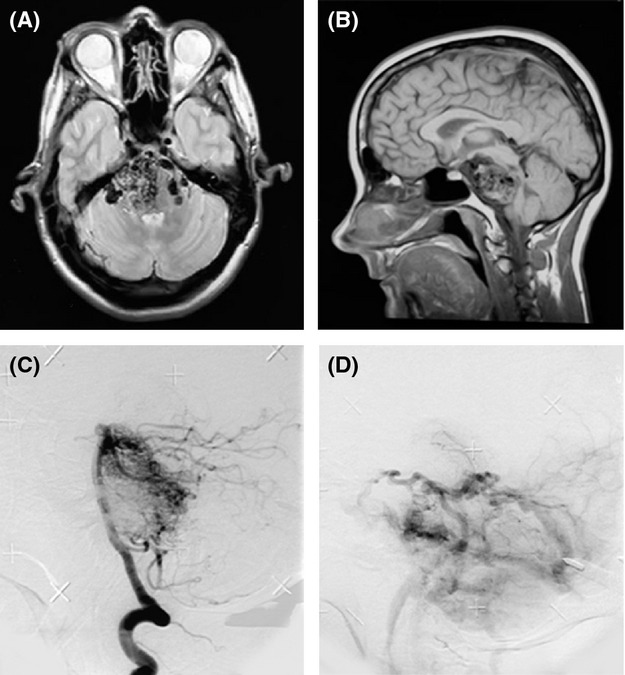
AVM located in right pons. (A) Axial T2-weighted MRI brain. (B) Sagittal T1-weighted MRI brain. (C) Digital subtraction arteriogram, vertebral artery injection, lateral view, arterial phase. (D) Digital subtraction arteriogram, vertebral artery injection, lateral view, venous phase. AVM, arteriovenous malformations; MRI, magnetic resonance imaging.
